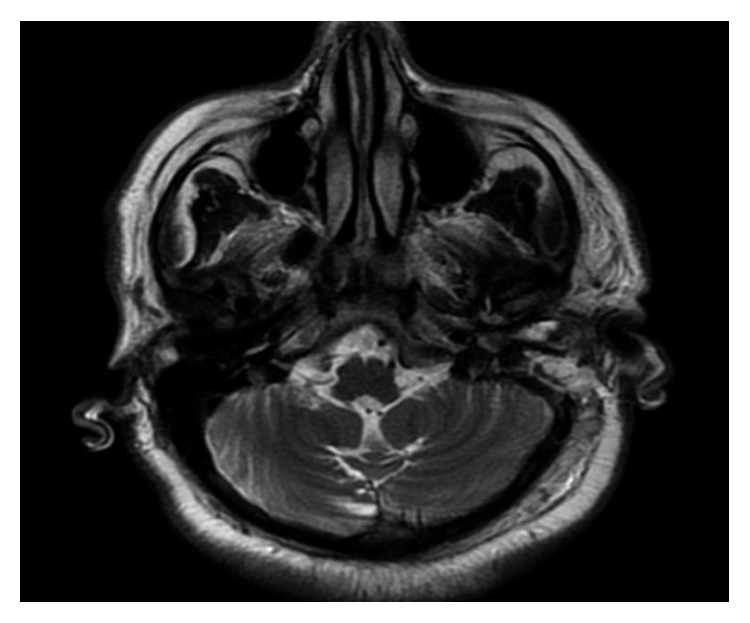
MRI of cerebellopontine angles and posterior fossa (axial cut). Opacification of the left mastoid air cells and middle ear cavity with soft tissue signal which elicits low signal in T1WI and high signal in T2WI with restricted diffusion in DWI. There is homogeneous enhancement following IV contrast administration. No evidence of adjacent intracranial abscess formation or sinus thrombosis. The region of brain stem and cerebellar hemispheres are normal. No focal lesions are seen. Both the VII and VIII cranial nerves show normal course and signal intensity. No intra- or extra-canalicular enhancing lesion is seen.
